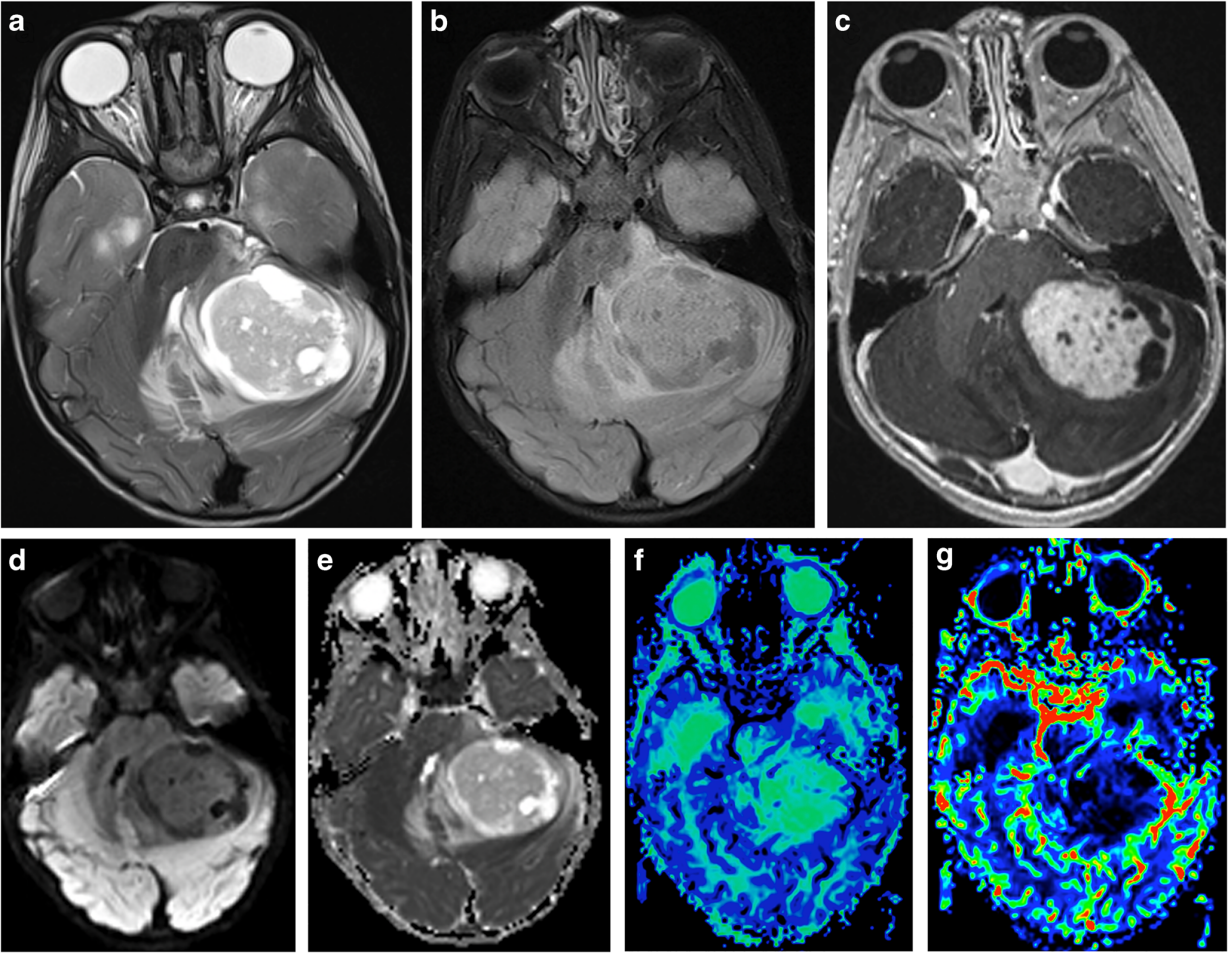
Pilocytic astrocytoma. a Axial T2-weighted scan through the cerebellum demonstrating a well-defined solid-cystic mass in the left cerebellar hemisphere that is heterogeneous onT2-weighted sequence and causes mass effect to the 4th ventricle. The solid component is minimally hyperintense compared to the gray matter. b An axial T2 FLAIR image through the same level better demonstrates the area of T2 hyperintensity beyond the tumor margin, suggestive of edema. c An axial post-contrast T1-weighted sequence through the same level demonstrates intense enhancement of the solid component of the tumor. d The axial diffusion image and ADC map (e) through the same level show no diffusion restriction. f The mean transit time map demonstrates increased transit time within the solid component of the tumor. g The cerebral blood volume map shows that the tumor has very low cerebral blood volume
